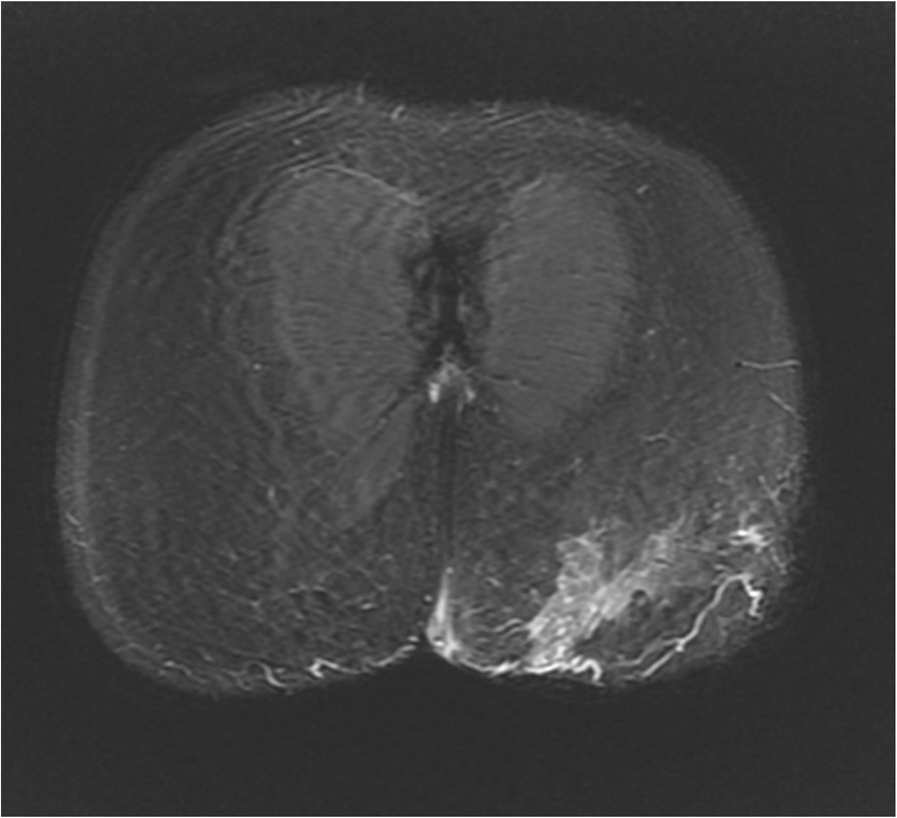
T1-weighted fat-saturated post-contrast magnetic resonance imaging showing pseudo-tumoral infiltration of the subcutaneous fat tissue in the left gluteal region (10 cm × 1.35 cm). Although the muscular plan is slightly involved, the infiltration does not reach a bony structure
